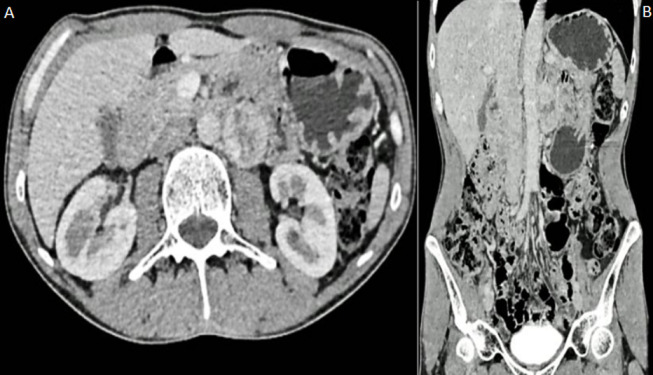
A) axial contrast-enhanced abdominal CT scan showing a left latero-aortic mass with heterogeneous enhancement; B) coronal contrast-enhanced abdominal CT scan showing the left latero-aortic mass
